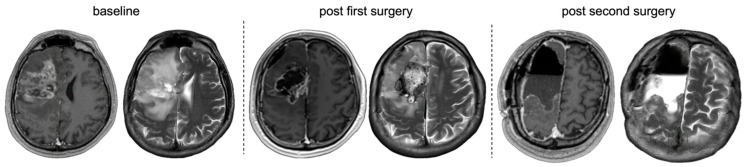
MRI from a representative case with non-compliance at first surgery and supramaximal resection after two-stage surgery. From left to right. T1-CE and T2-nCE baseline to post second surgery. Postoperative MRI after first awake surgery, right frontal demonstrating T1-CE as well as T2-nCE residual tumour. MRI after the second surgery shows no residual T1-CE or T2-nCE tumour.
